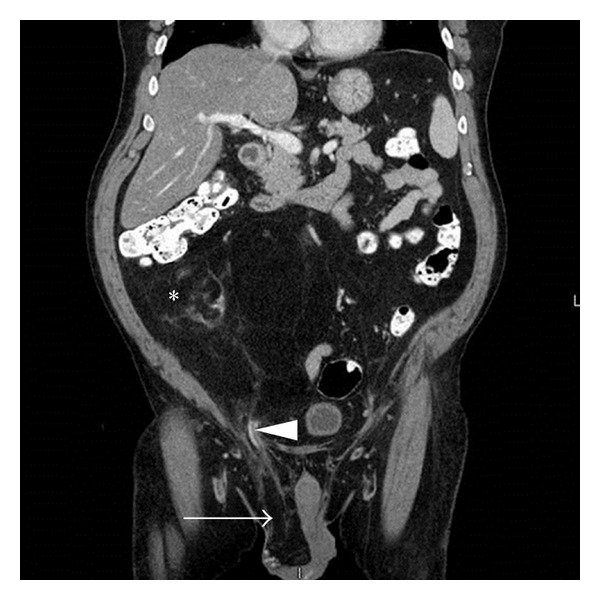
CT abdomen and pelvis with IV contrast, coronal image, demonstrating a large fatty lesion with associated soft tissue component in the right peritoneum extending into the right lower quadrant along the right paracolic gutter measuring 10.3 × 7.4 cm and 18.1 cm in superior to inferior direction. The lipomatous lesion extends into the inguinal canal (white arrowhead) resulting in right-sided inguinal hernia (white arrow). There is an area of ill-defined soft tissue component (starred). Bowel loops are displaced to the left.
